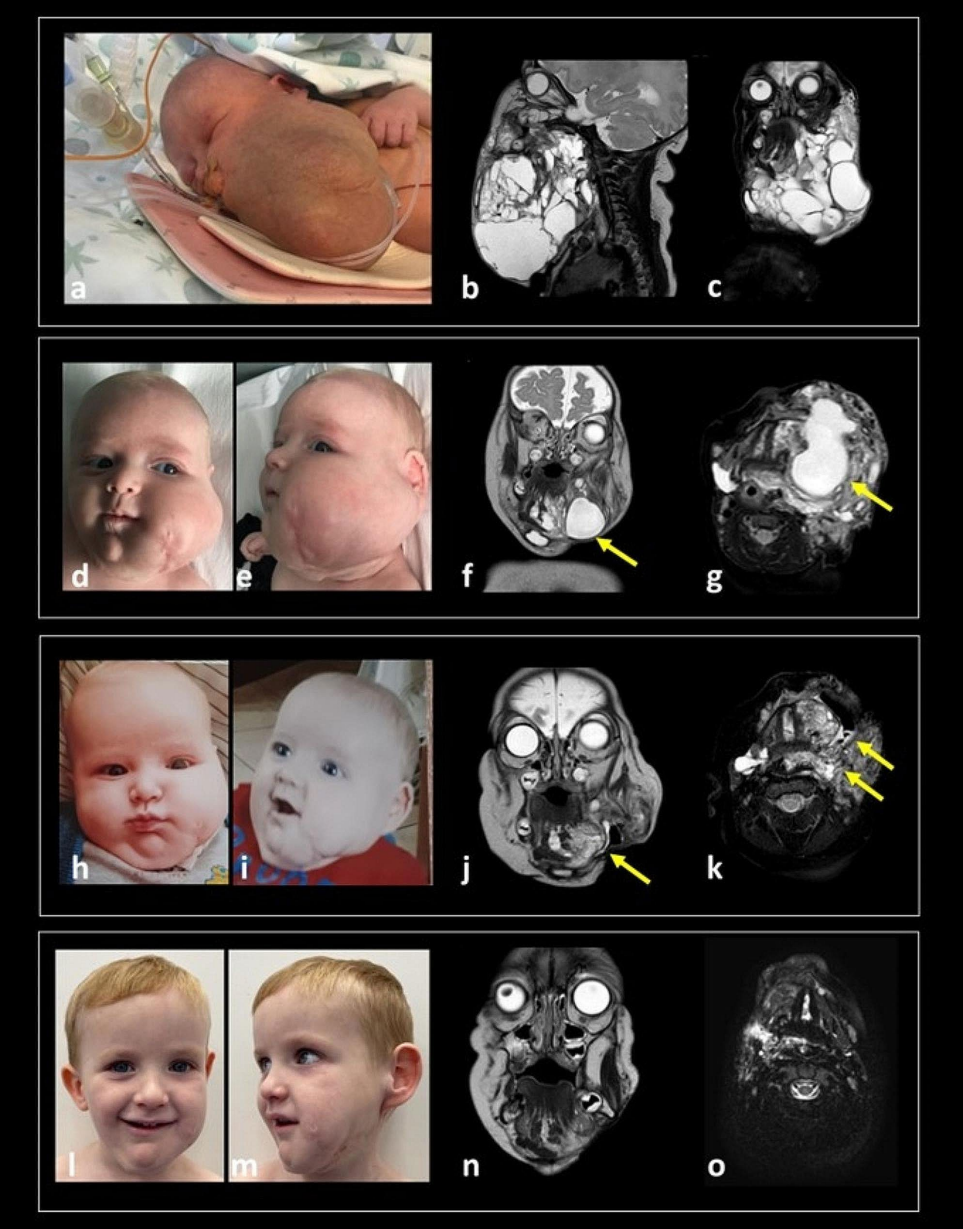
A large mass with elastic consistency involving the neck and the left side of the face was confirmed at birth(a). MRI at the time showed a large multicompartmental mixed solid-cystic mass (mixed solid enhancing and cystic non-enhancing components) that involved the left face and the suprahyoid and infrahyoid neck superficial and deep spaces, including parapharyngeal and visceral spaces infiltration (b, c). At 3 months of life, a clinical rapid worsening of the swelling mass in the left cheek was detected (d, e). A follow-up MRI (f, g) confirmed increased volume of some macrocystic components along the left submandibular and parapharyngeal spaces (arrows). After the second surgical procedure, a clinical improvement of the facial asymmetry was evident with reduction of the left swelling cheek mass (h, i) and the MRI (j, k) showed residual microcystic components in the left sublingual and parapharyngeal spaces (arrows). At last evaluation, 4 years 6 months old (l, m), head and neck MRI (n, o) revealed a reduction of the residual lymphatic tissue located in the left sublingual and submandibular spaces and in the left neck (Left MRI column: sagittal T2-w; Right MRI column: coronal T2-w)
